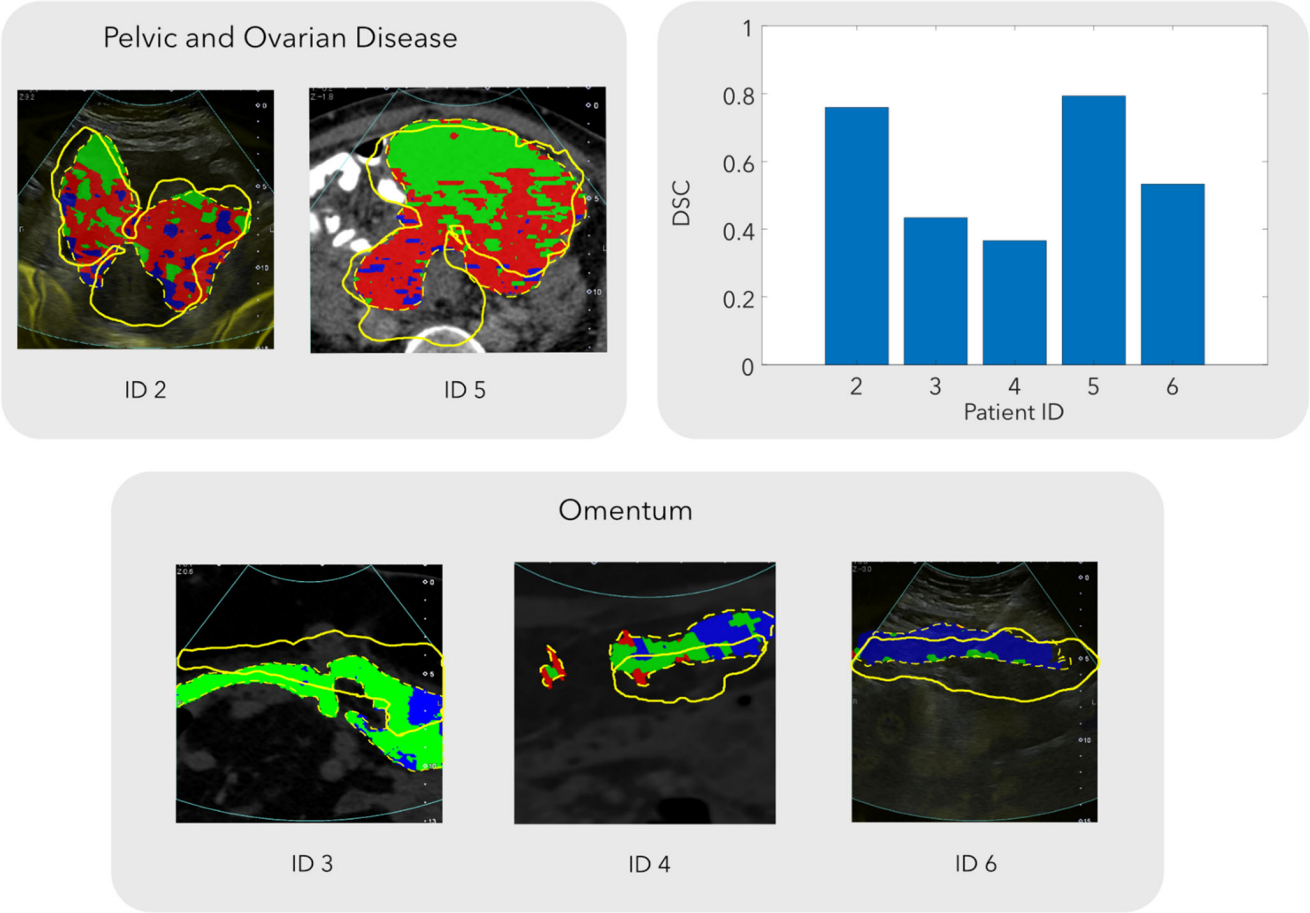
The accuracy of the CT/US fusion was associated with the tumour volume and was higher for pelvic compared to omental tumours. The dice similarity coefficient (DSC) was used to assess the accuracy of the CT/US fusion. The DSC was higher for the pelvic (larger) compared to that for the omental (smaller) tumours that yielded a low DSC. Dashed line, CT segmentation; solid line, US segmentation
